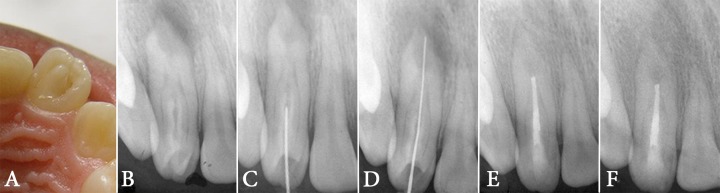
A) *The protuberated cingulum in the palatal aspect of the left maxillary lateral incisor, *B)* Periapical radiolucency around the apex of the laterla incisor with *type III* dens in dente, *C) *Entrance into the lumen without involving the main canal, *D) *Working length determination, *E) *Obturation of the lumen and *F) *Follow-up image after 18 months; the periapical lucency has disappeared
